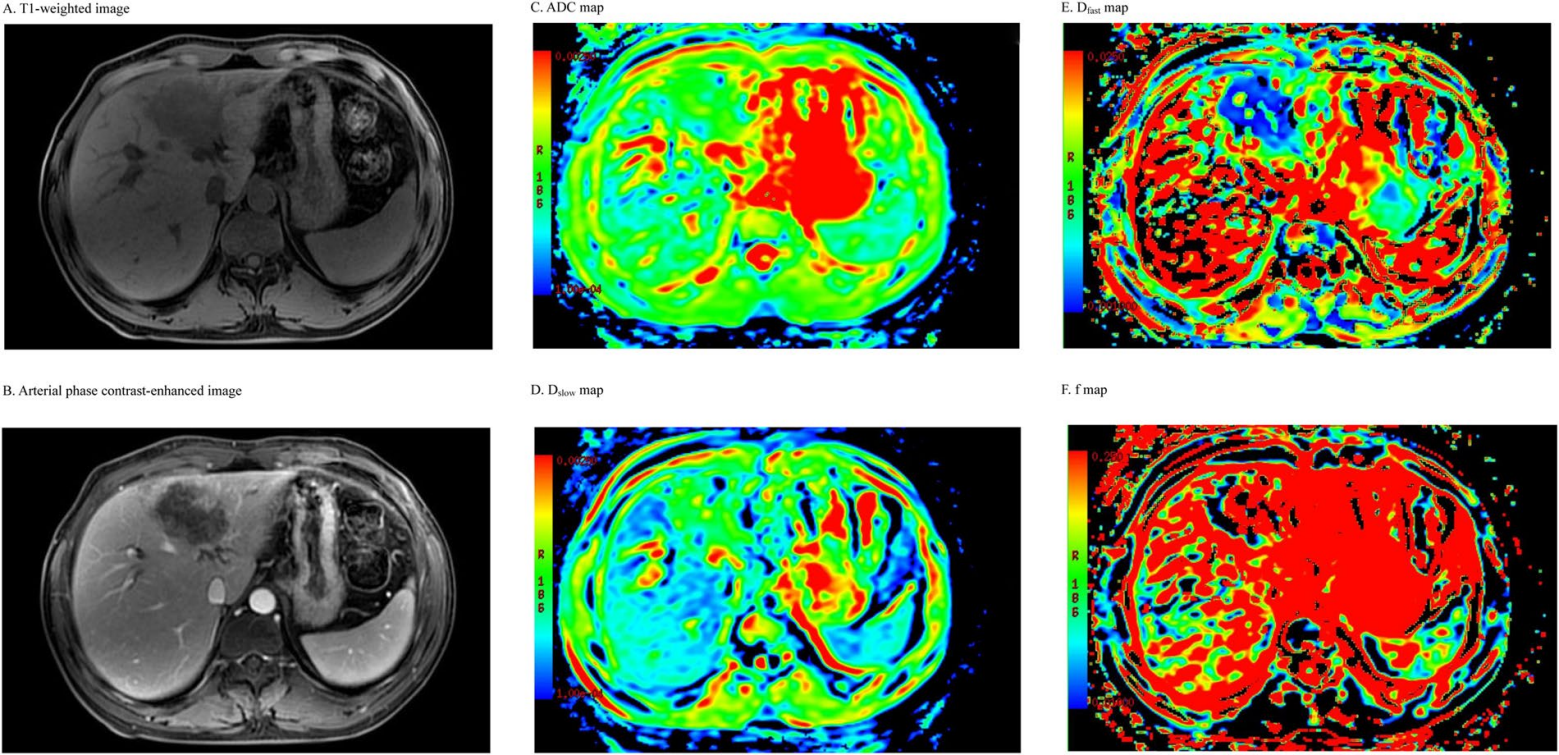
MR images of an ICC patient. (A): A T1-weighted image shows a hypointense lesion in the left lobe of the liver. (B): An arterial phase contrast-enhanced image shows an inhomogeneous enhanced lesion with biliary obstruction. (C): ADC map. (D): D_slow_ map. (E): D_fast_ map. (F): f map.
